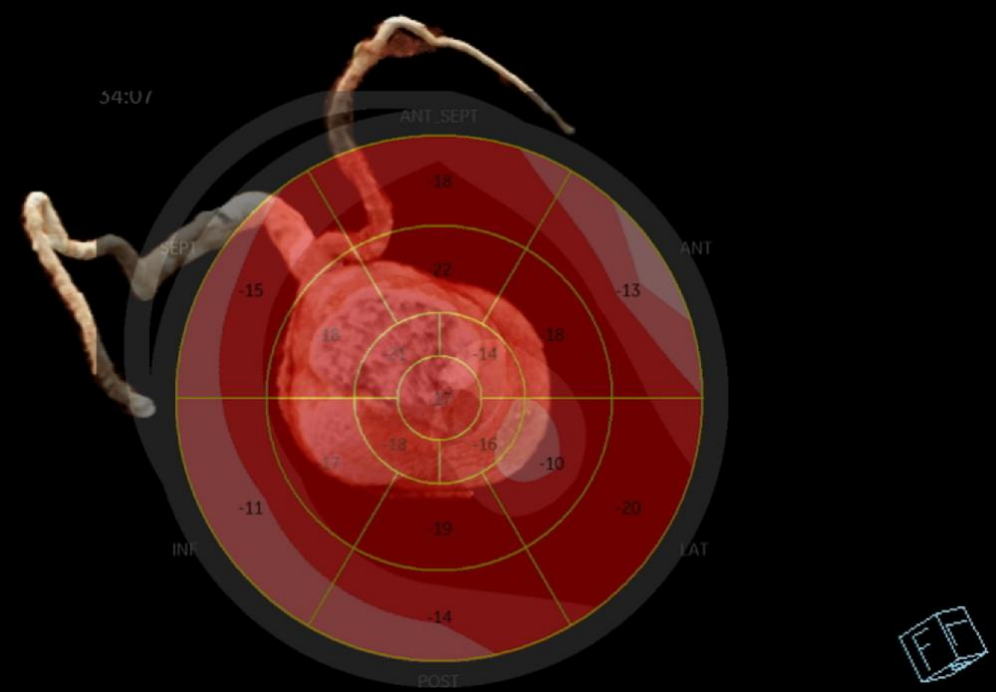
Fusion of strain echocardiography (‘bulls eye’) and computed tomography angiography images.
